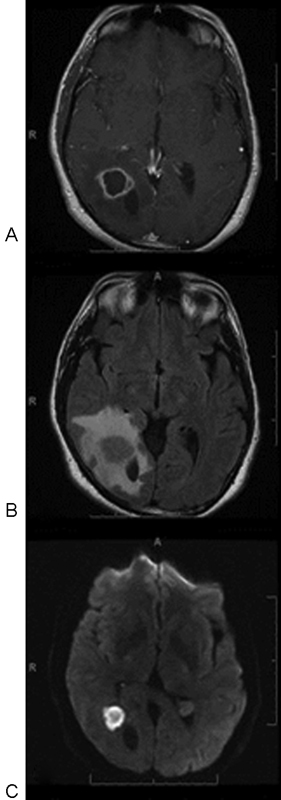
(A) Axial T1-weighted postgadolinium magnetic resonance imaging showing an enhancing cystic lesion adjacent to the right occipital horn of the lateral ventricle. (
